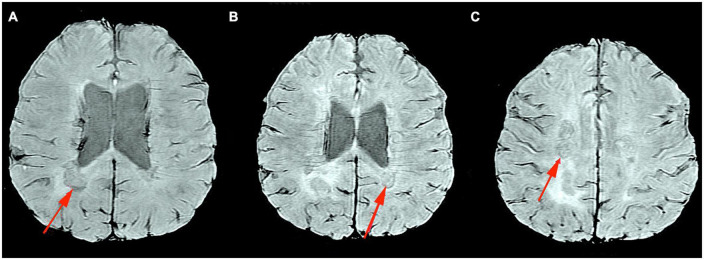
MRI Swan sequence, axial section. Iron rim sign in a patient with SPMS (A–C). Material from the resources of the Medical Radiology Department of the Military Medical Institute–National Research Institute.
